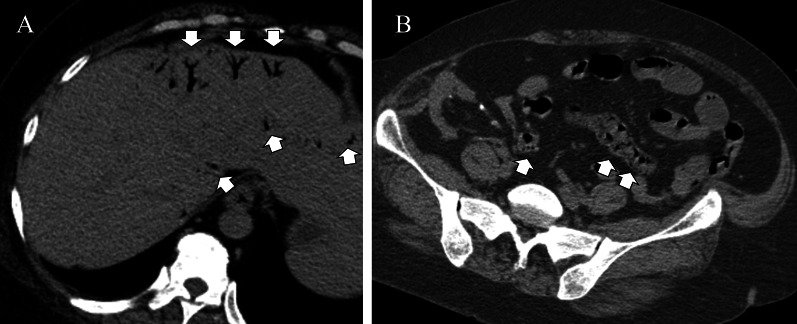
A Plain abdominal CT reveals portal gas (arrows). B CT also showed emphysema in the small intestine (arrows) and ascending colon
